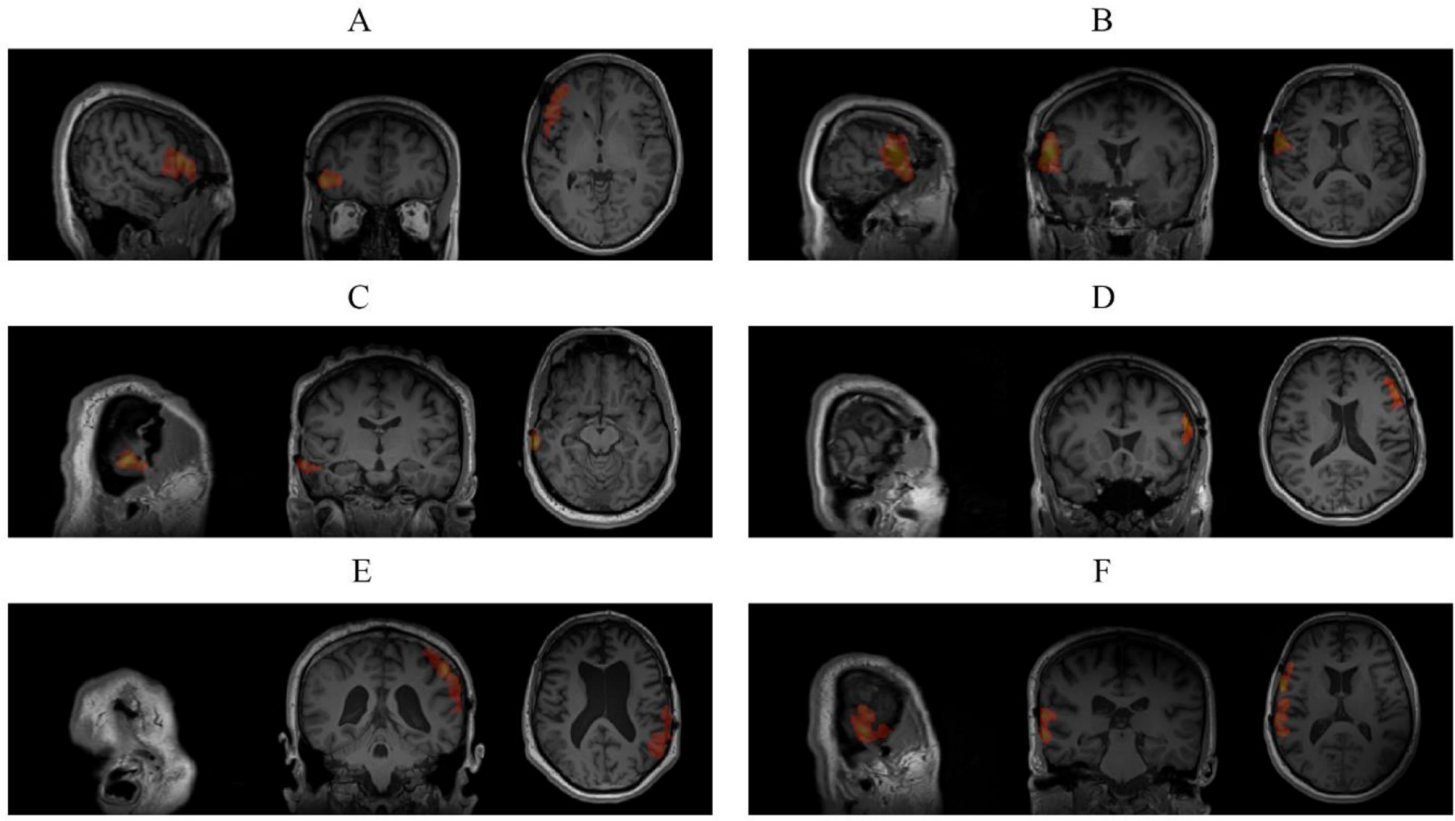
The post-surgical MRI with overlaid localized SOZ cluster on resection area using the presented method: (A) subject 1 with behavioral arrest with oral automatisms and verbalization showing rhythmic alpha and theta activity at right temporal lobe (T4>F8) in ictal EEG; (B) subject 2 with bilateral limb automatisms showing rhythmic theta activity at both temporal lobes (T>F) but right irritative zone in ictal EEG; (C) subject 5 with left versive motion and left limb dystonia showing rhythmic alpha activity at right temporal lobe (T4>T3) in ictal EEG; (D) subject 6 with staring with oral automatisms, right versive motion, and right facial clonic activity showing rhythmic theta activity at left temporal lobe (T3>T4) in ictal EEG; (E) subject 7 with behavioral arrest, left limb automatism, and right versive motion showing rhythmic theta activity at left temporal lobe (T3>T4) in ictal EEG; (F) subject 8 with behavioral arrest with staring.
